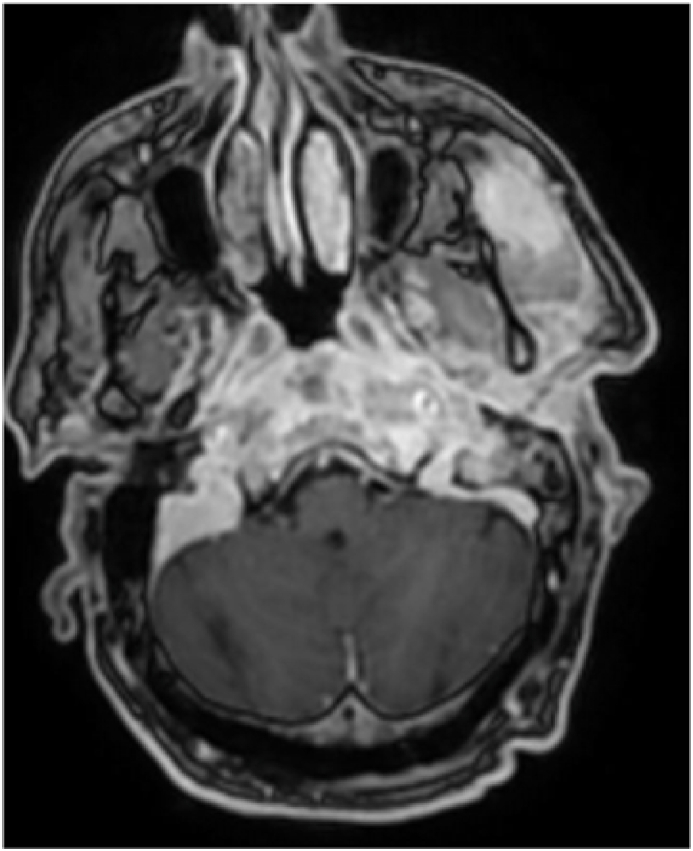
Cervical MRI showing infiltration and enhancement of the inner ear extended to the deep spaces of the face related to malignant otitis externa.
